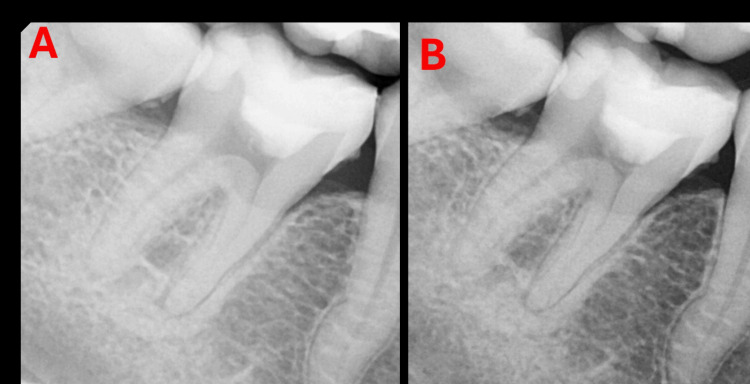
Radiographic follow-up. (A) Immediate postoperative radiograph. (B) Eleven-month follow-up showing healing.
